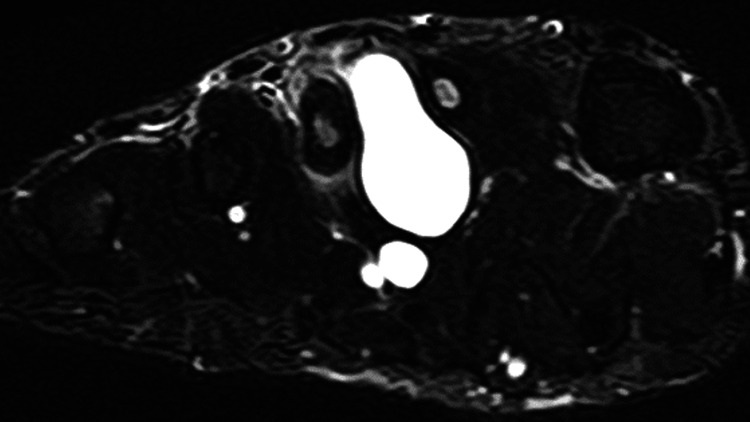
MRI axial image showing gourd-shaped cysts between the second and third metatarsals.
